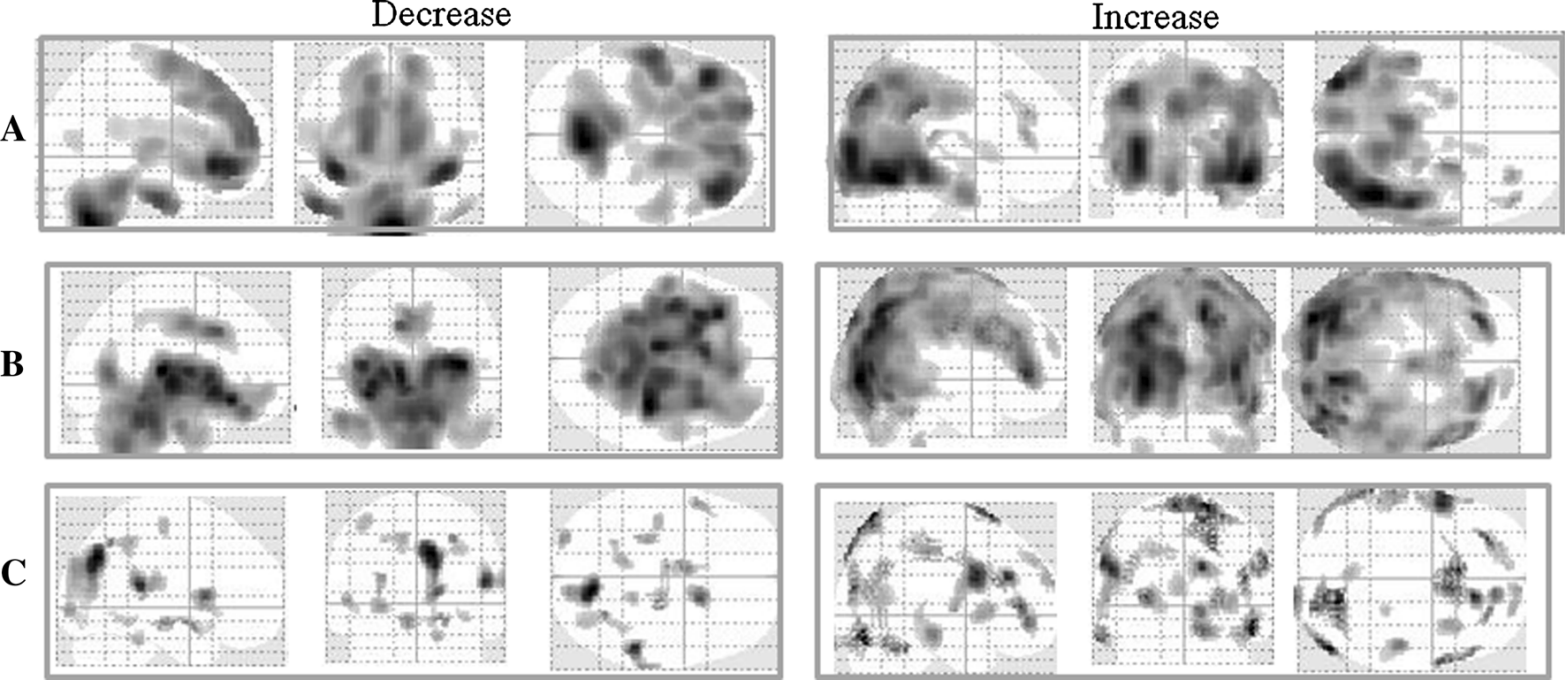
Maximum intensity projection (MIP) of SPM8 result for each two-sample *t* test on basis of E.CAM Ref PAO data by ^99m^Tc-HMPAO SPECT. MIPs show the increase and decrease for IRIX data (a), IRIX AC^−^ data (b), and compensated IRIX AC^−^ data by Normal-SPECT (c), respectively
